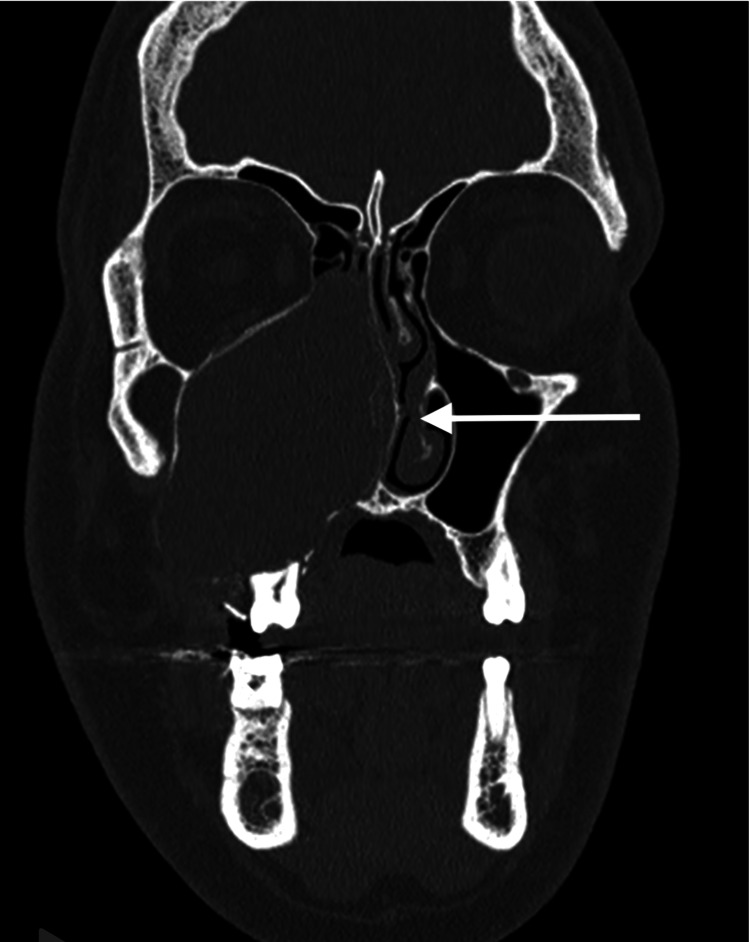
Coronal CT of the paranasal sinuses (bone window) Coronal CT scan demonstrating complete opacification and expansion of the right maxillary sinus with thinning and remodeling of the medial and palatal bony walls, extending toward the nasal cavity. CT: computed tomography
